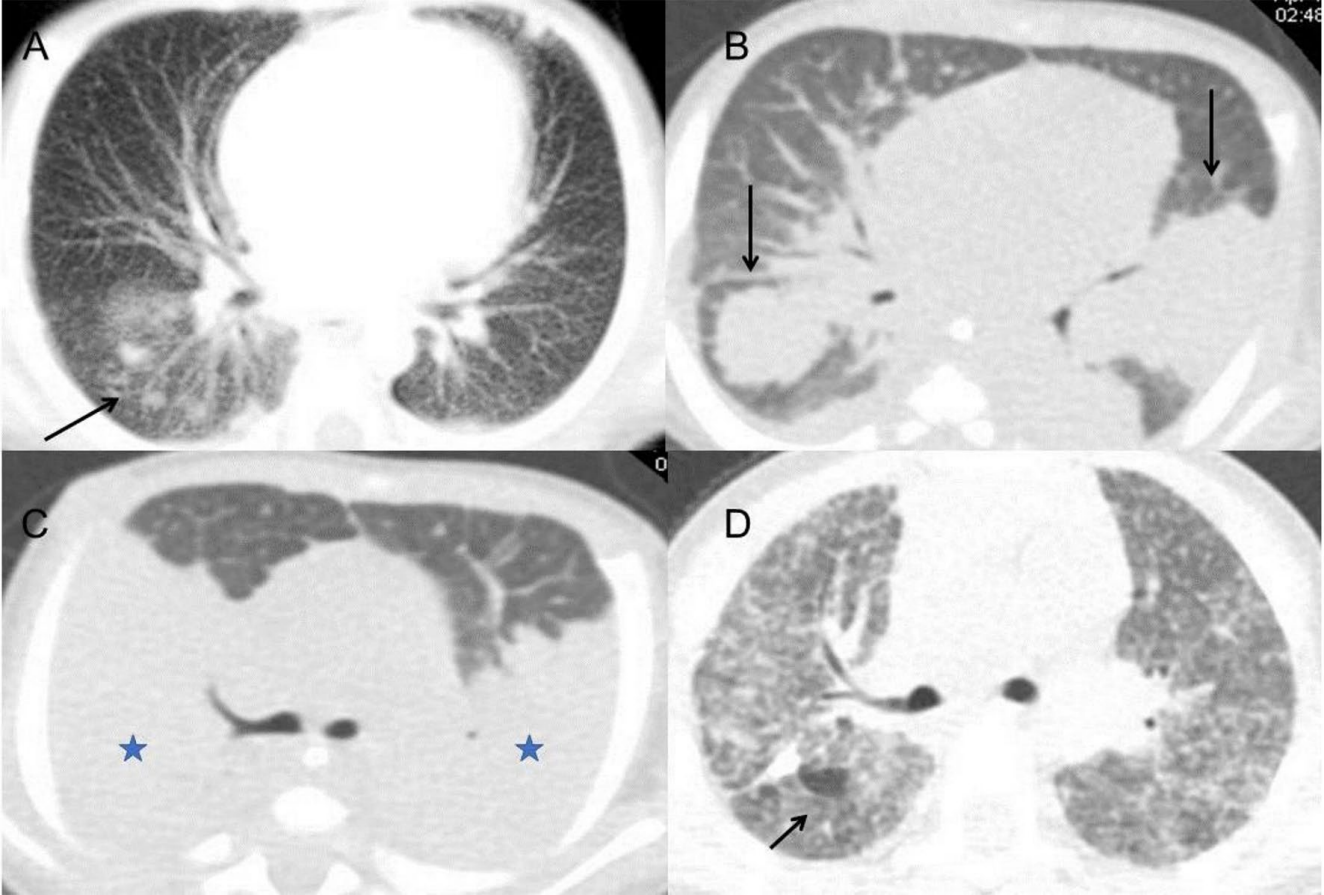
CT images of fungal infection. (A,B) Multiple nodules (A, long arrow) and masses (B, long arrow) were found in the bilateral lower lobes on axial CT images. (C) Chest CT showed bilateral large areas of consolidation (star) in a 1-year-old boy. (D) In a 2-year-old boy, extensive interstitial pneumonia with tree-in-bud opacities, interlobular septal thickening and paraseptal emphysema (short arrow) were demonstrated.
